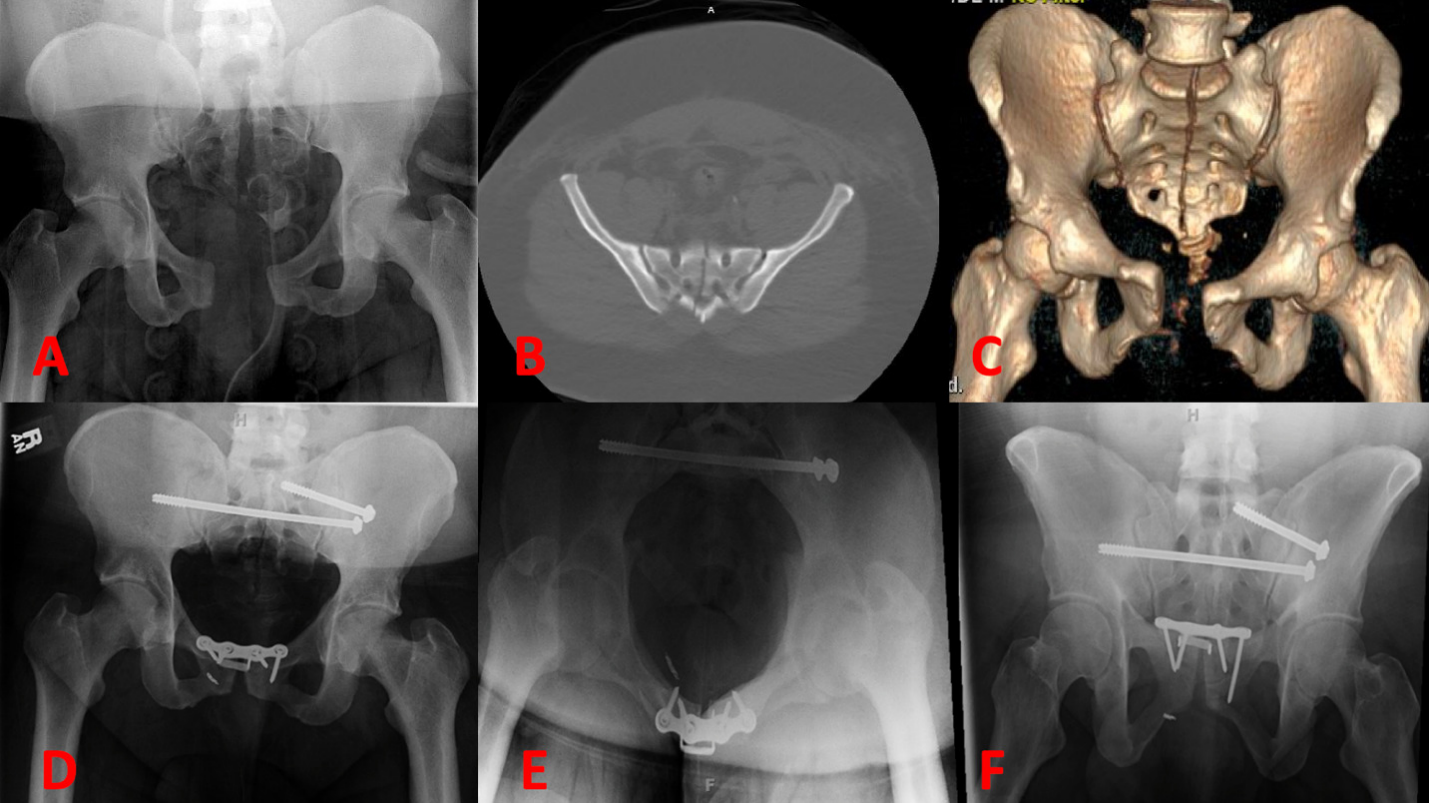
A complete anterior to posterior midline longitudinal sacral fracture as seen on (A) Anterior-Posterior (AP) Radiograph, (B) Axial cut of CT Pelvis (bone window), (C) 3D reconstruction, and post-operative (D) AP, (E) inlet, and (F) outlet radiographs.
